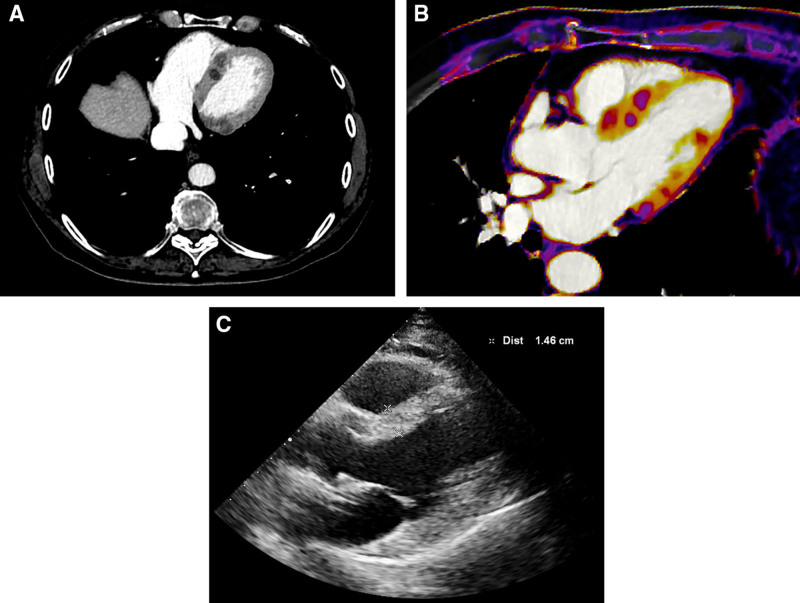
Computed tomography and transthoracic echocardiography findings. (A) Axial contrast-enhanced total-body computed tomography image performed during portal venous phase; (B) multiplanar image reconstruction along the 3-chamber long-axis view with iodine map overlay. Both images show multiple hypodense lesions within the myocardium, with extensive involvement of inferior and septal segments; (C) transthoracic echocardiography image along parasternal long-axis view reveals only septal hypertrophy.
